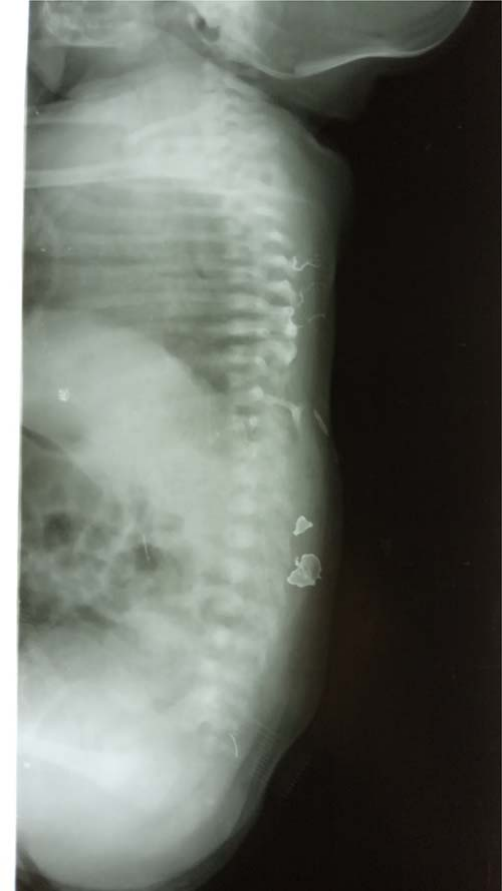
Lateral abdominal x-ray showing the defected vertebra.
